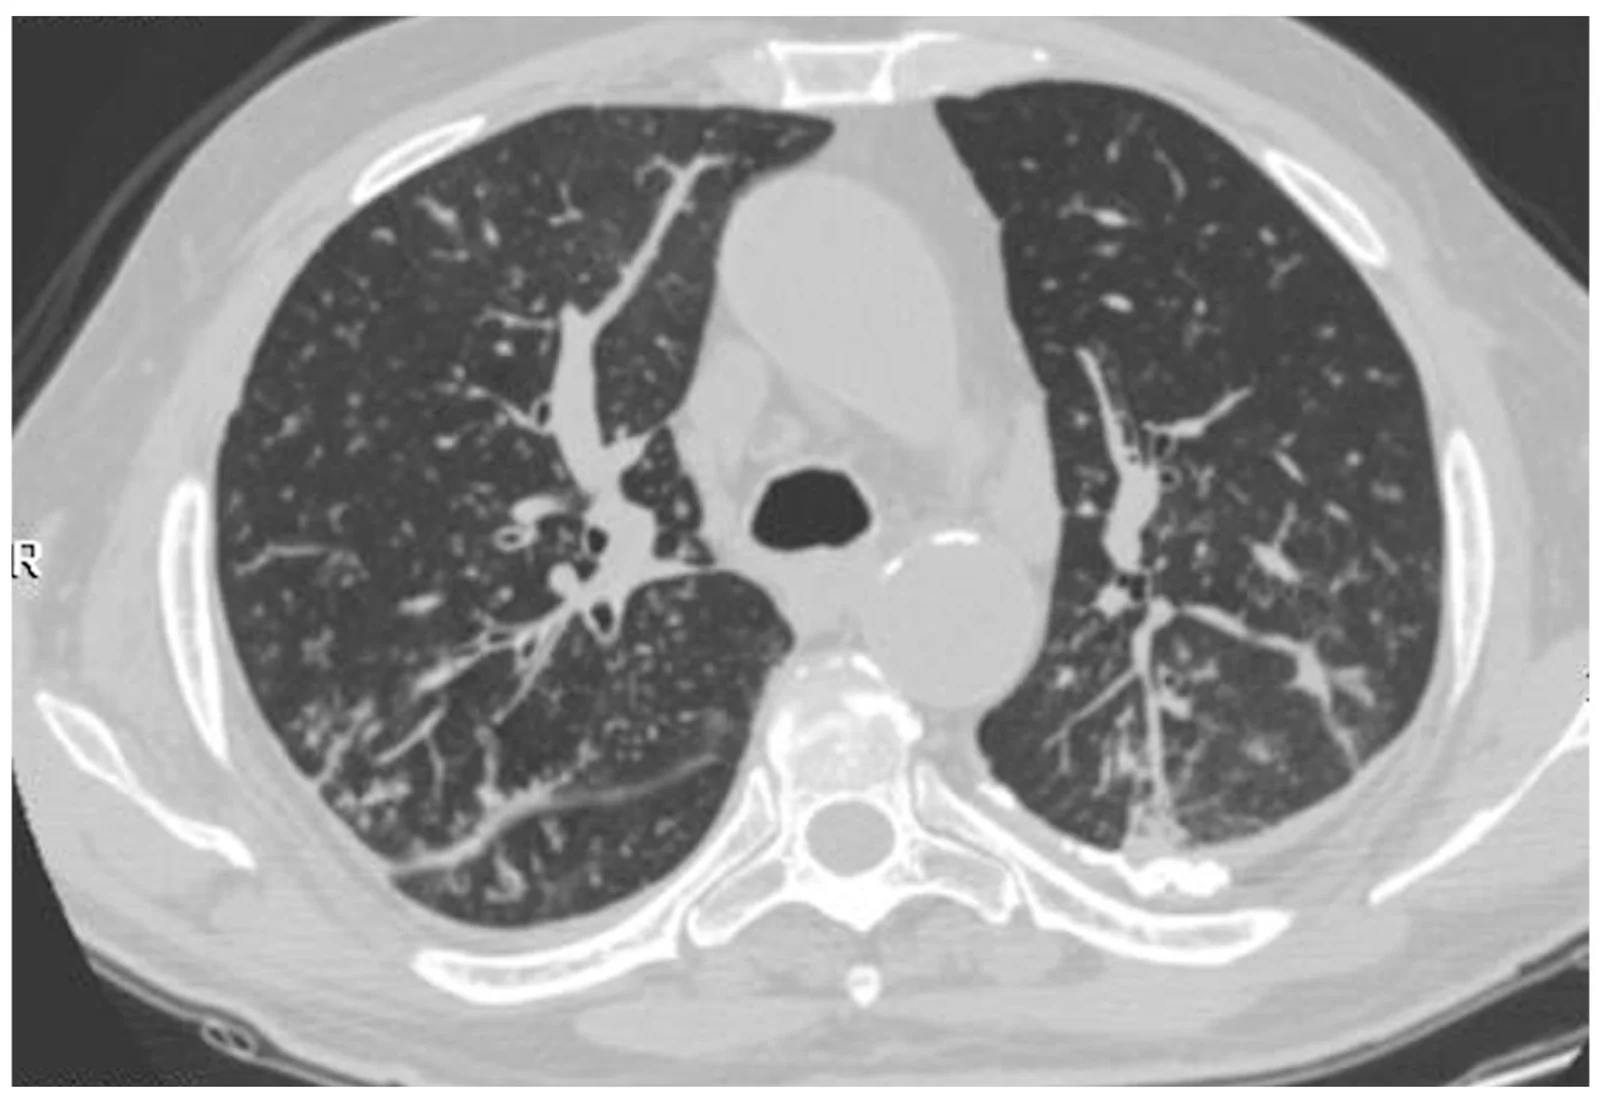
HRCT, axial plane. CT scan shows the nodular bronchiectatic form of MAC infection in a male patient, although this pattern is more frequent in female patients. In this case, there is a HRCT tree-in-bud pattern, with involvement of the distal airways and numerous centrilobular micronodules in the right upper lobe and lingula. This radiological pattern is more uncommon in the bacterial GLDs but has to be included in the differential diagnosis of other type of infectious and non-infectious bronchiolitis.
